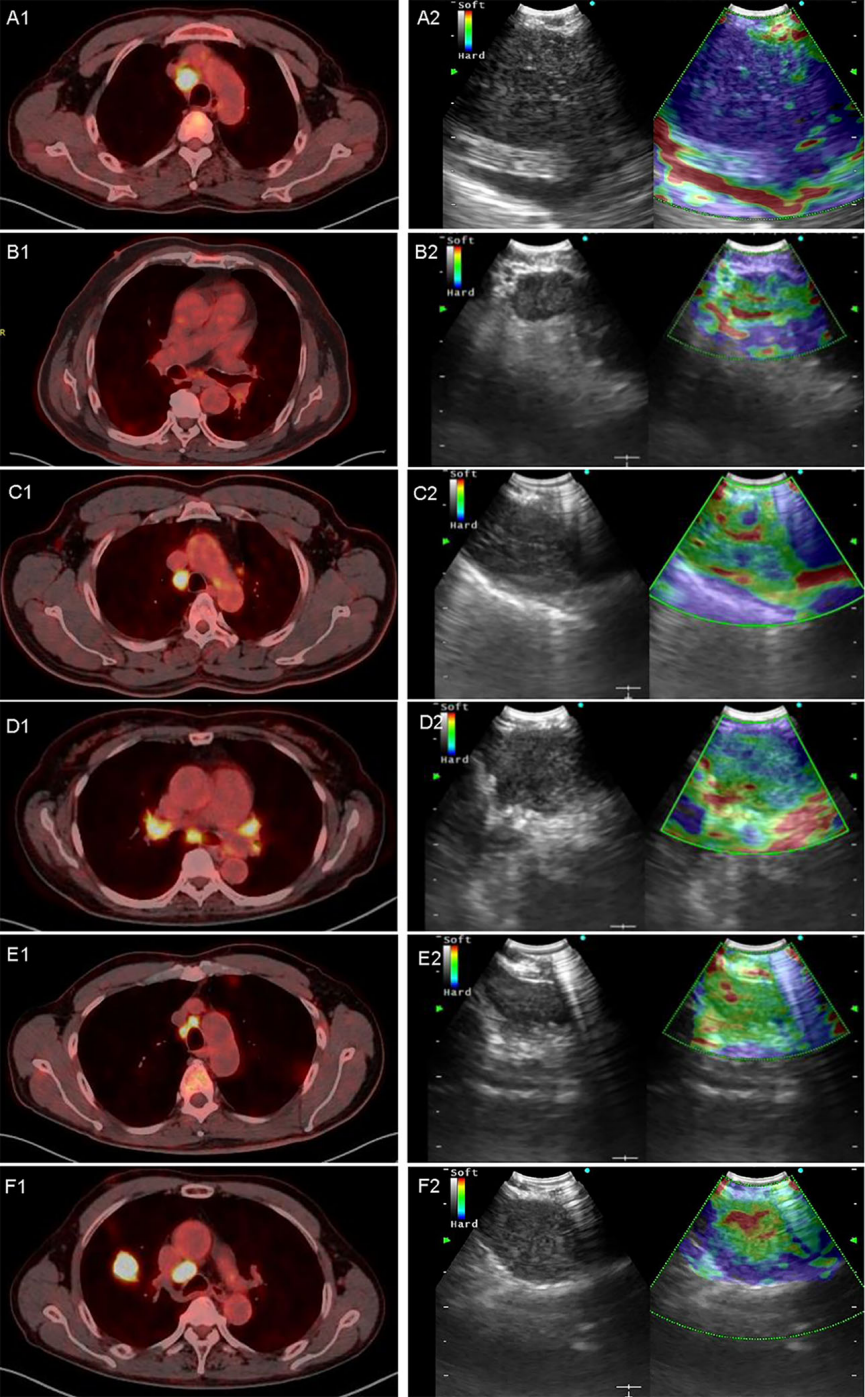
Representative images of PET/CT and elastography in a variety of diseases. A1 and A2 are representative true-positive images of malignant LNs examined by PET/CT and elastography, which showed a 4R LN with adenocarcinoma; B1 and B2 show a 7 LN with nonspecific lymphadenitis, true-negative results in both elastography and PET/CT; C1 and C2 show a 4R LN with tuberculosis, and PET/CT showed high metabolism with an SUVmax of 7.85 and a TLG of 39.09. In contrast, elastography showed a grading score of 3 and a mean hue value of 117.74; D1 and D2 showed a 7 LN with sarcoidosis, in which PET/CT showed a high metabolism while elastography showed soft tissue. SUVmax, TLG, grading score, and mean hue value were 5.5, 22.06, 3, and 105.84, respectively; E1 and E2 showed a 4R LN with nonspecific lymphadenitis, false positive by PET/CT but true negative by elastography, and SUVmax, TLG, grading score, and mean hue value were 7.84, 11.33, 1, and 89.37, respectively; F1 and F2 show a 4R LN with neuroendocrine tumor not otherwise specified, and PET/CT showed high metabolism with an SUVmax of 13.29 and a TLG of 42.19. However, elastography shows false-negative results with grading score of 3 and mean hue value of 124.42. LN, lymph node; SUVmax, maximal standardized uptake value; TLG, tumor lesion glycolysis.
